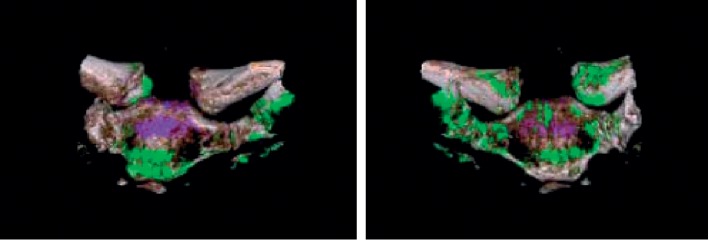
Sternoclavicular details with Dual Energy CT 3D reconstruction.
